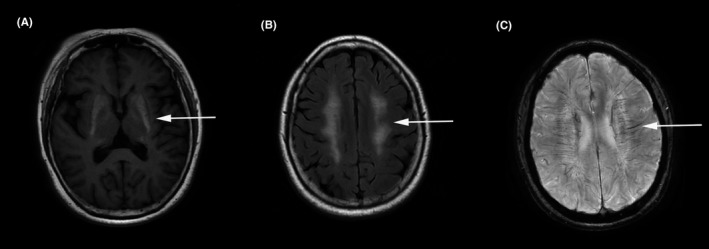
Brain magnetic resonance imaging showed lesions in the bilateral basal ganglia with short T1 signals (A, arrow), lesions across the bilateral centrum semiovale with long T2 signals (B, arrow), as well as slight dilations of the deep medullary vein along the bilateral periventricles on susceptibility weighted imaging (C, arrow)
